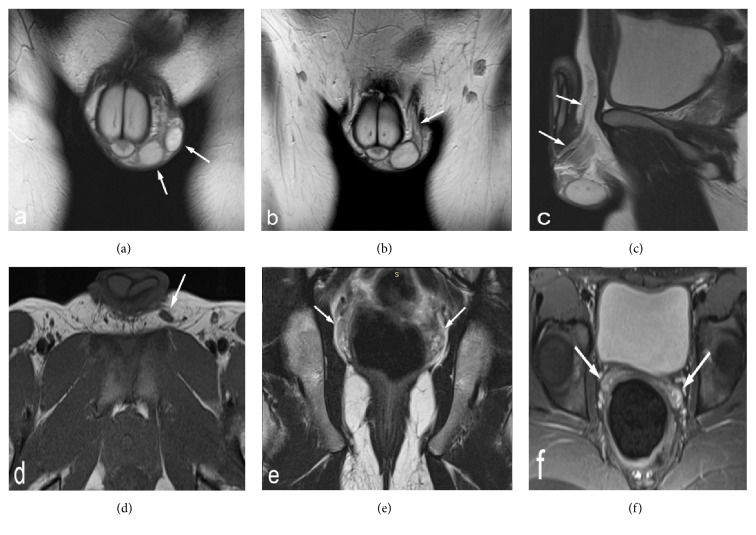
MR images of the scrotum and pelvis. (a) Coronal T2-w image reveals two testes in the left hemiscrotum, as ovoid-shaped masses with high signal intensity (arrows). (b, c, and d) Coronal T2-w, sagittal T2-w, and axial T1-w images demonstrate the single fused vas on the left side surrounded by fatty tissue (arrows). (e, f) Coronal T2-w and axial T2-w with fat suppression (FS) images depict the hypoplastic seminal vesicles with intermediate to high signal intensity (arrows).
